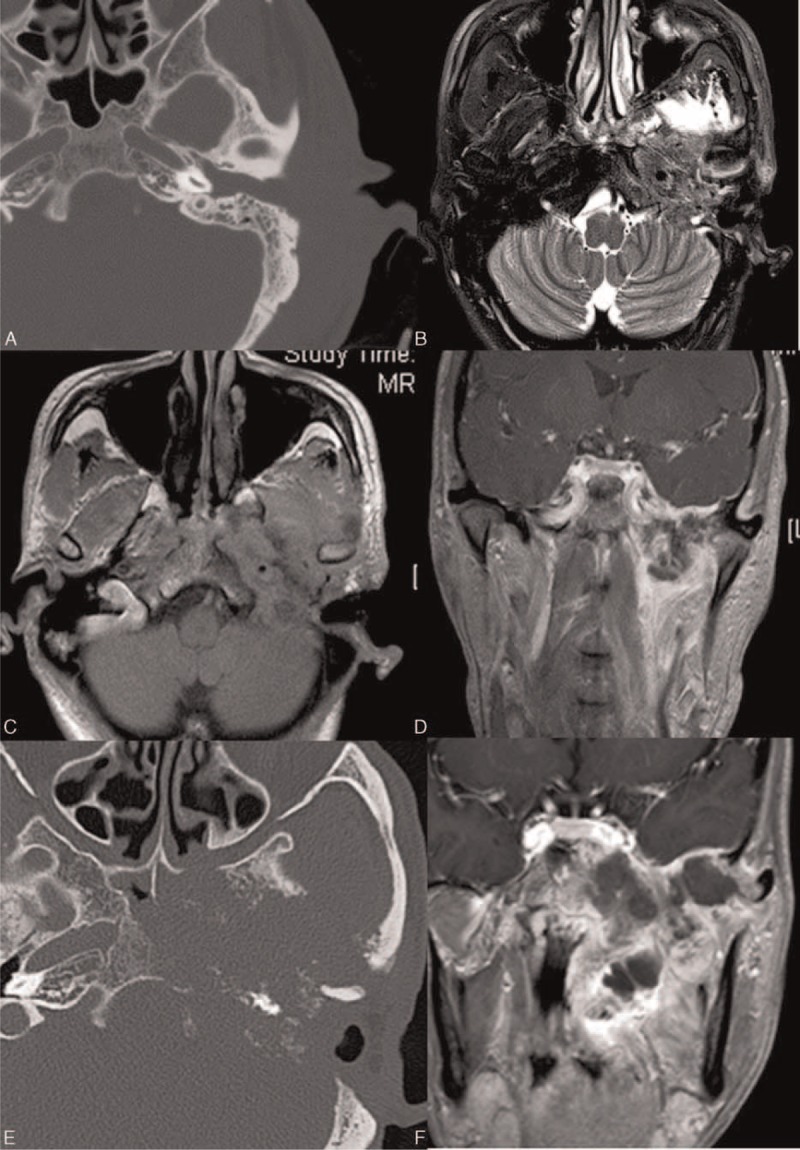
A 48-year-old man (case 1, SCC, stage 4) with complaints of otalgia and otorrhea of left ear for 1 month which worsened over last 5 days before presentation. Axial HRCT and MRI were done. He died 7 months later due to the recurrence. A large soft tissue mass is seen in the left EAC combined with anterior and posterior wall destruction (A). The lesion extensively involves the parapharyngeal space, subcutaneous area, encasing the sigmoid sinus, jugular fossa, and carotid artery without clear margin (B, C). Dura is also involved on contrast-enhanced MRI and the lesion shows heterogeneous enhancement (D). Lateral temporal bone resection was performed and pathologically confirmed to be SCC. Six months later, the patient complained of severe otalgia, fever and palsy of the cranial nerve III, IV, V IX, X, and XI. HRCT (E) shows extensive moth-eaten pattern of bone destruction including petrous bone, occipital clivus, sphenoid body, and carotid canal. Necrosis is noted on contrast enhancement MRI (F). EAC = external auditory canal; HRCT = high resolution computer tomography; MRI = magnetic resonance imaging; SCC = squamous cell carcinoma.
